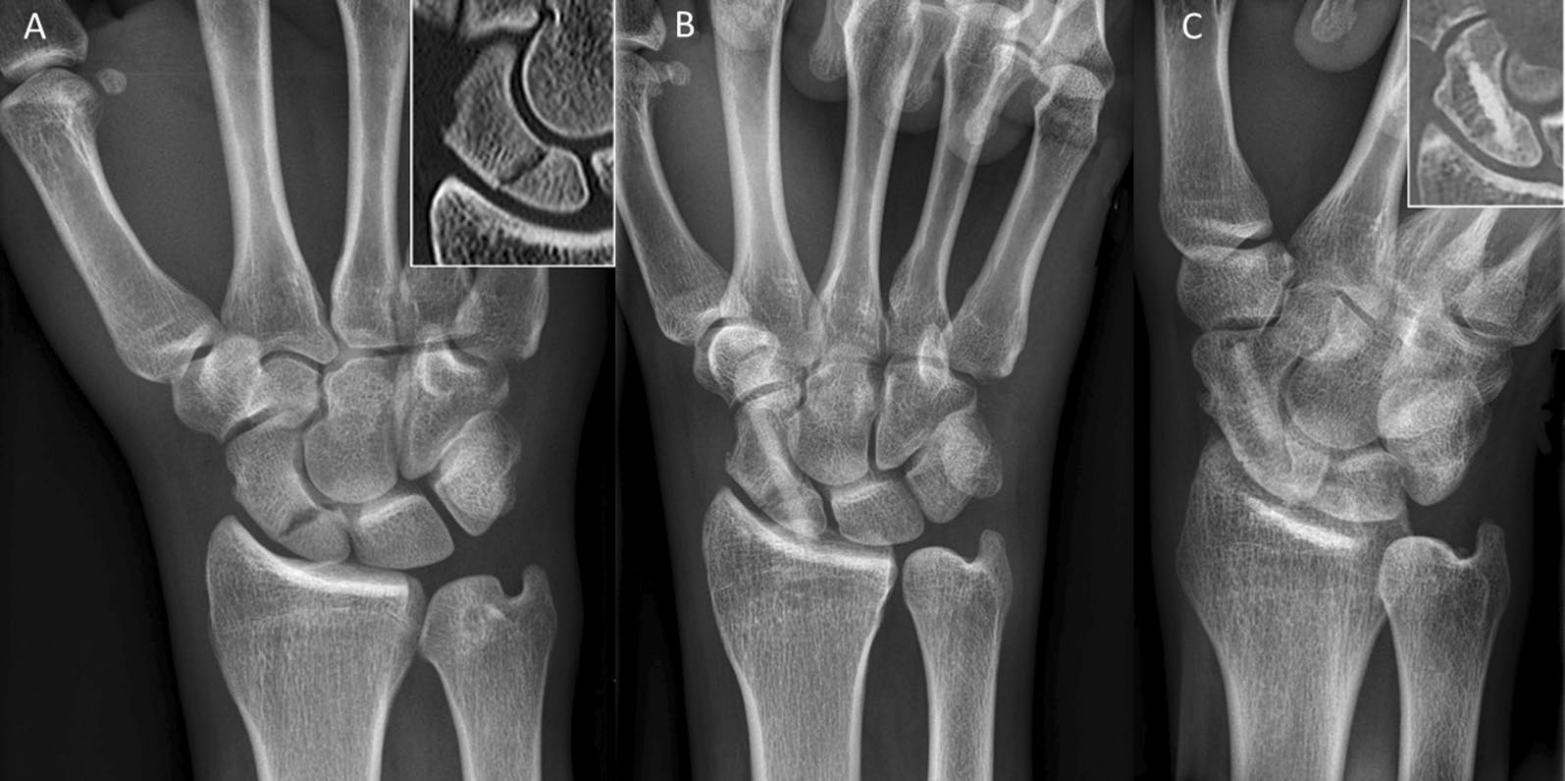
Pseudarthrosis of the scaphoid in the proximal pole. A Pre-surgery,* Inset*: CT of the scaphoid. B 1 day post-surgery. C 10 months post-surgery.* Inset*: CT of the scaphoid
